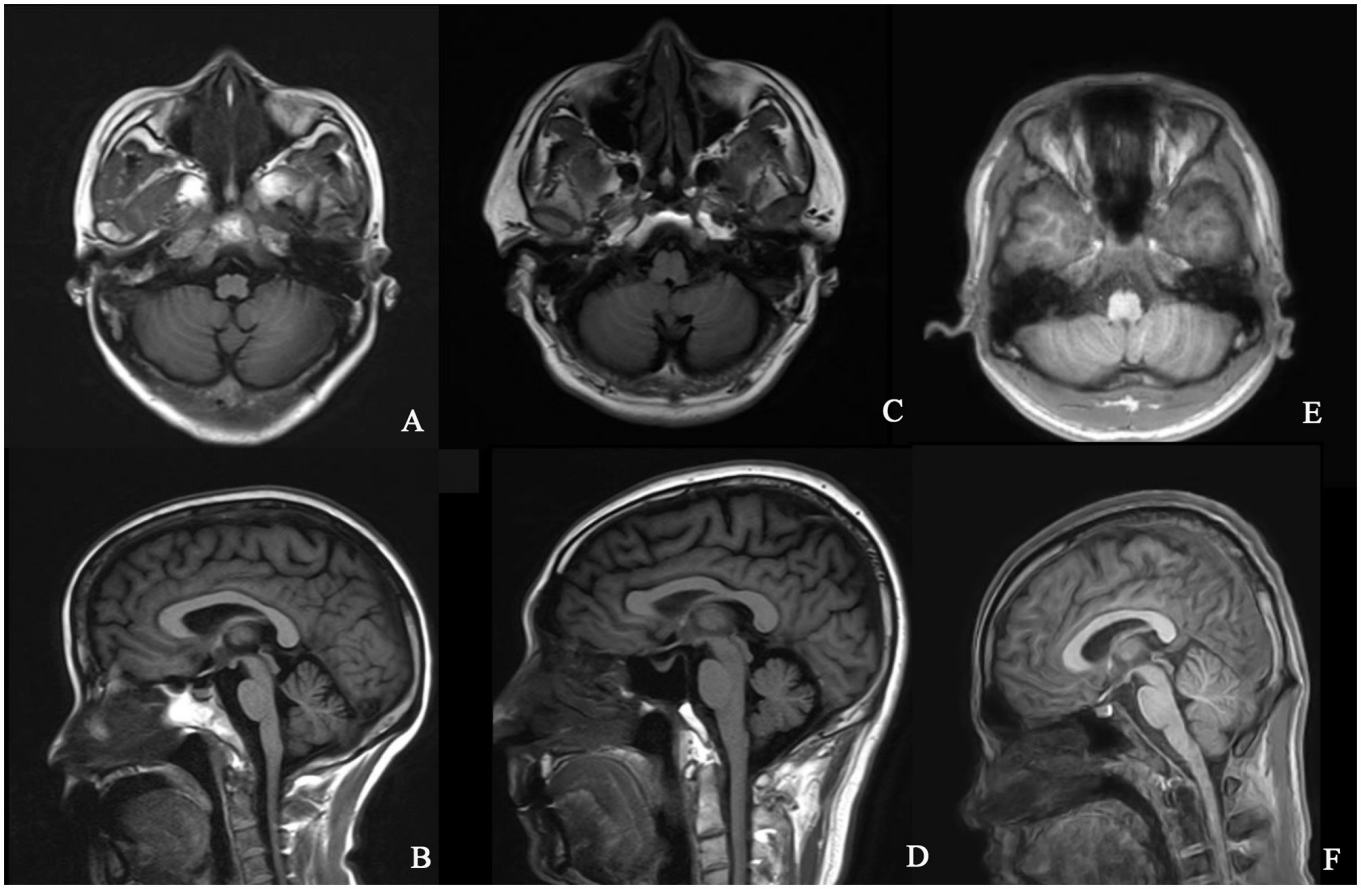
Brain MRI in patients with SCA3, SCA17, and SCA28. T1 axial and sagittal images showing cerebellar atrophy in a 40-year-old female patient with SCA3 (A,B). T1 axial and sagittal images showing cerebellar atrophy in a 50-year-old male patient with SCA28 (C,D). No specific sign of cerebellar atrophy was found in T1 axial and sagittal images of a 17-year-old male patient with SCA17 (E,F).
